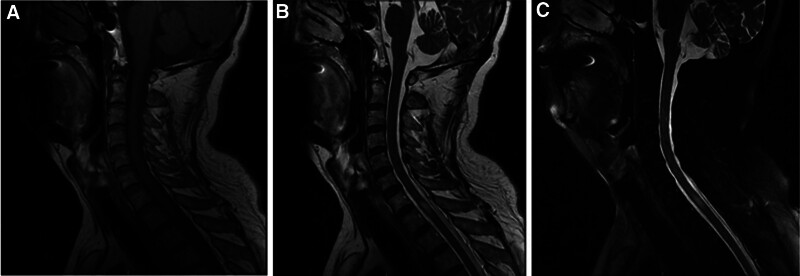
MRI indicated lesions of the spinal cord at T1 level. (A) T1WI. (B) T2WI. (C) T2 fat-saturation sequence. MRI = magnetic resonance imaging.
